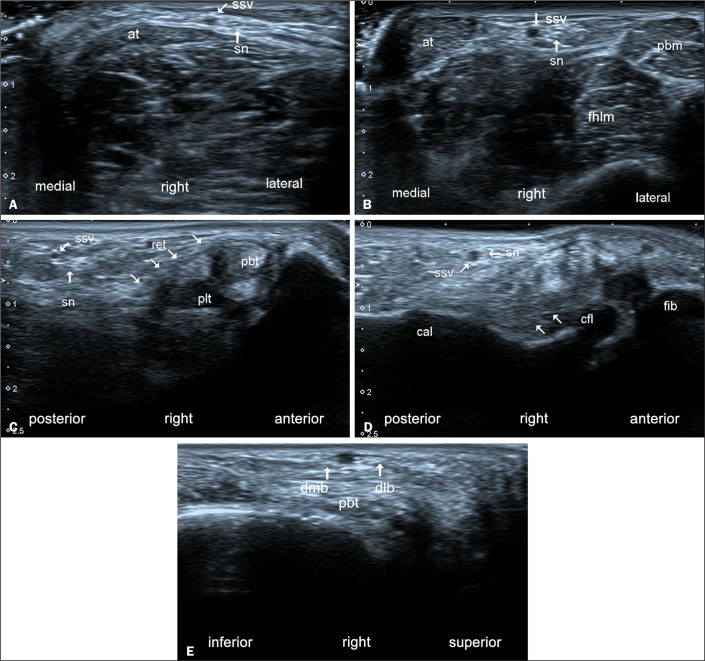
Ultrasonography in the axial plane (A,B) and in the coronal oblique plane
